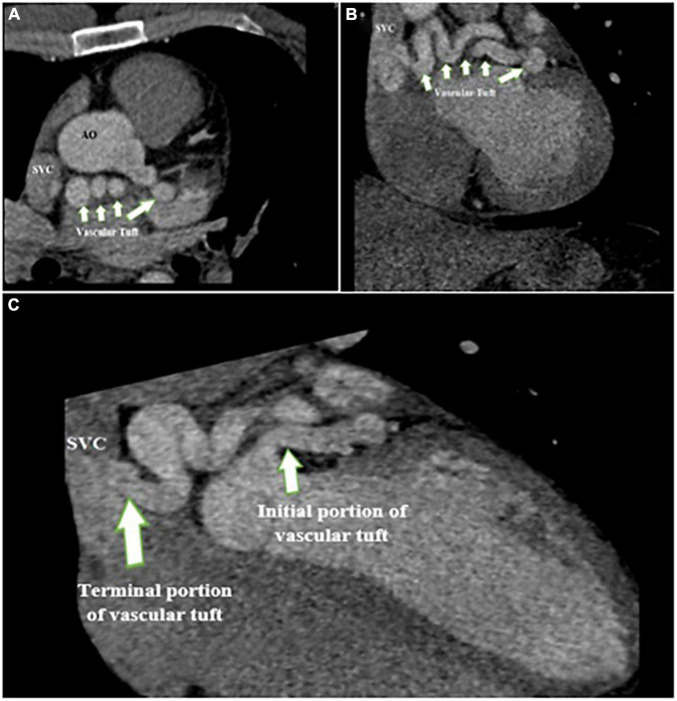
Contrast-enhanced CT scan of the chest shows the proximal site of giant fistula (white arrow) originating from the left circumflex artery with drainage into the superior vena cava.
